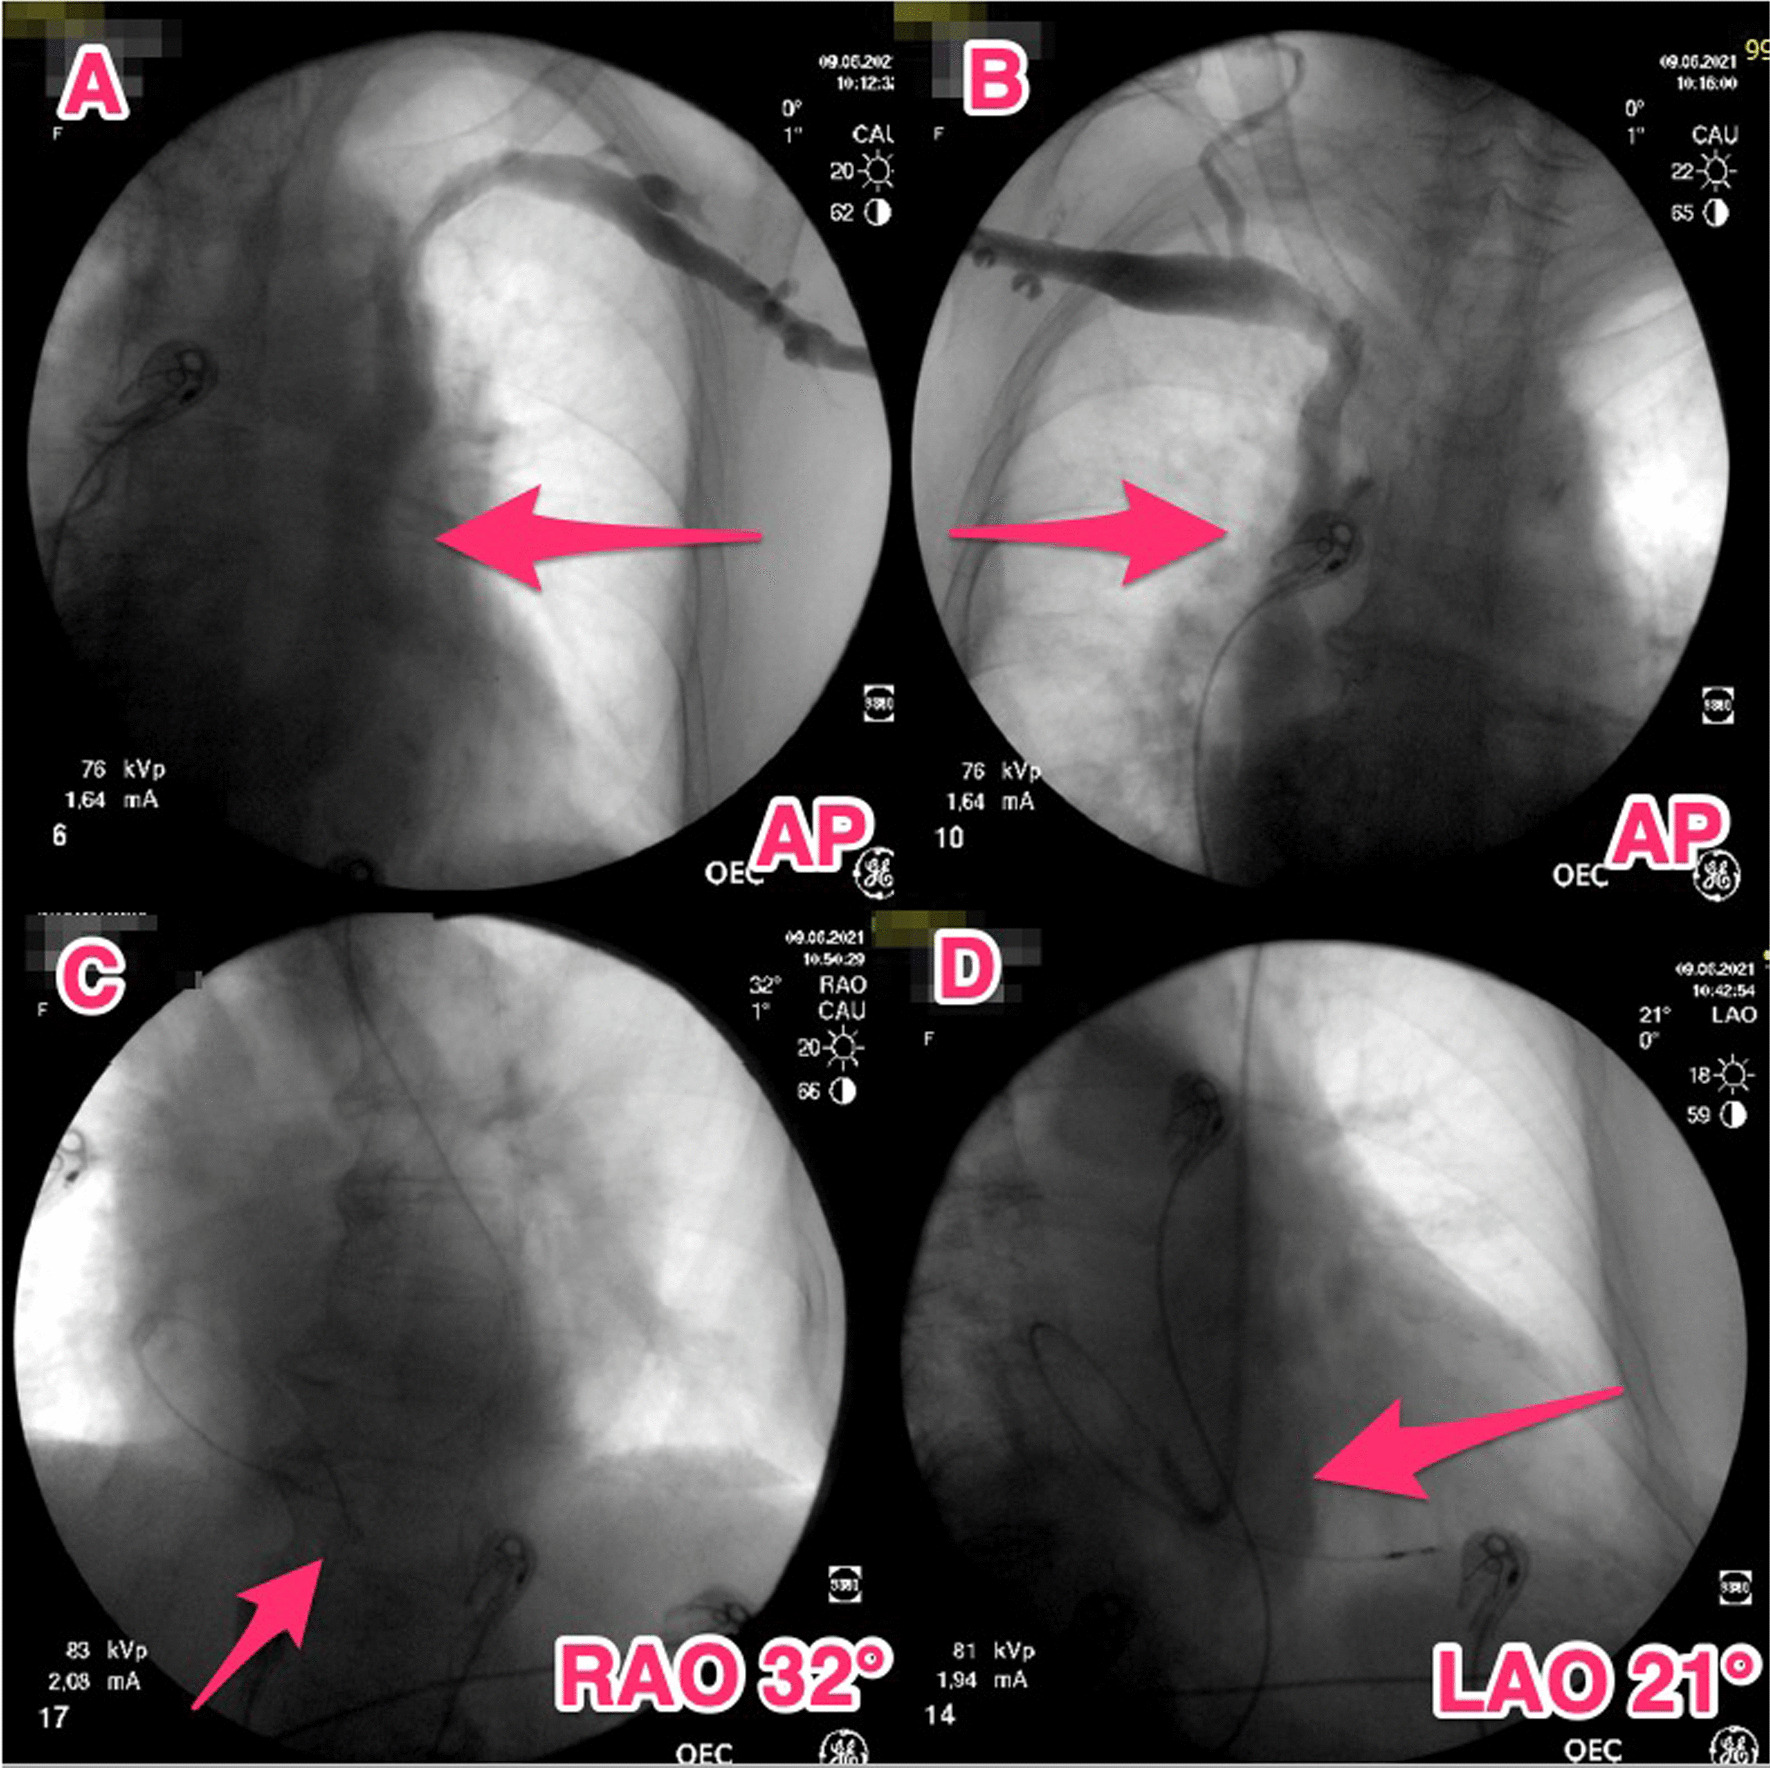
Chest X-ray. A posteroanterior projection; contrast visualization of persistent left superior vena cava (arrow); B posteroanterior projection; contrast visualization of absent right superior vena cava, subclavian vein draining into the azygous vein; right anterior oblique projection of cardiac pacing lead (arrow); D left anterior oblique projection of cardiac pacing lead in „alpha “ loop contouring the shape of coronary sinus
